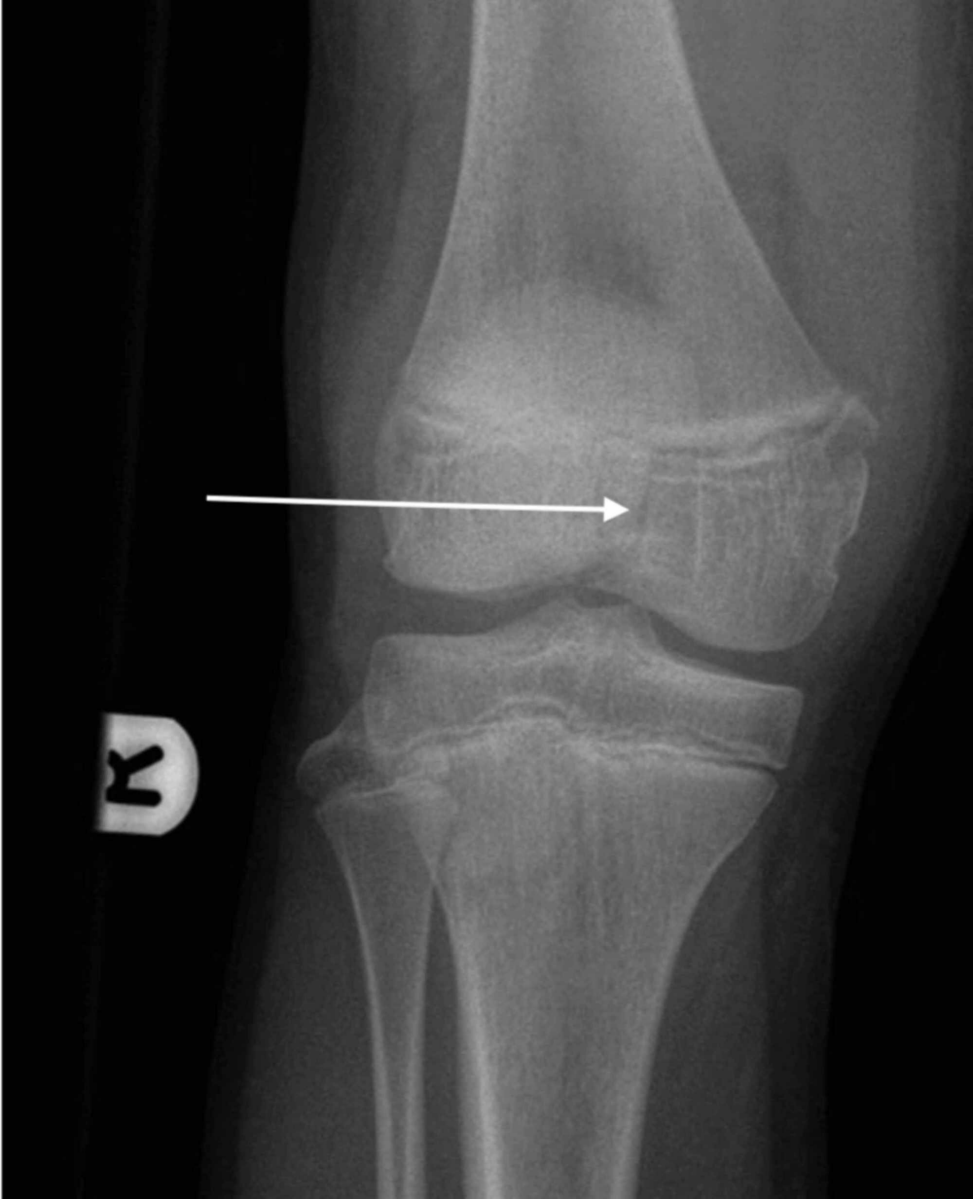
Anteroposterior x-ray of right knee post-injury
